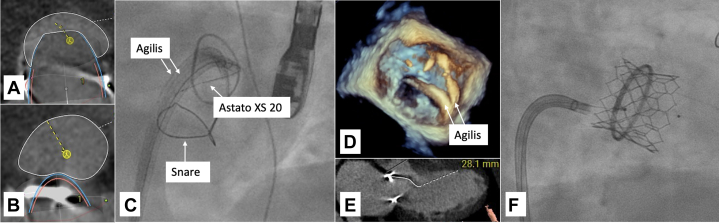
Imaging for Case 1 (A) CTA of neo-LVOT 207 mm^2^ without LAMPOON. (B) CTA rendering of neo-LVOT 388 mm^2^ with LAMPOON. (C) Fluoroscopy of antegrade LAMPOON. (D) Three-dimensional TEE of antegrade LAMPOON. (E) Anterior mitral leaflet length 28.1 mm. (F) Fluoroscopy showing valve-in-ring TMVR with 26-mm Edwards S3 at the time of subsequent paravalvular leak closure. CTA = computed tomography angiography; LAMPOON = laceration of the anterior mitral leaflet to prevent outflow obstruction; LVOT = left ventricular outflow tract; TEE = transesophageal echocardiogram.
